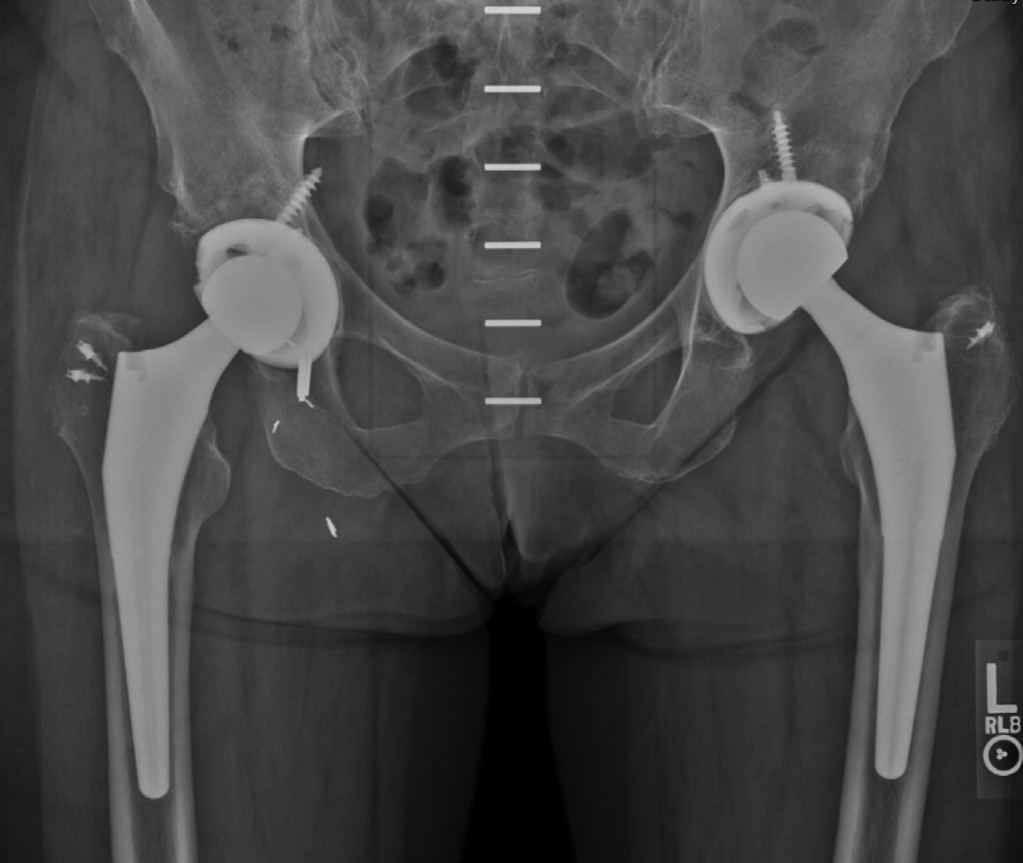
Postoperative pelvic radiograph after bilateral revision total hip arthroplasty, March 2021.
